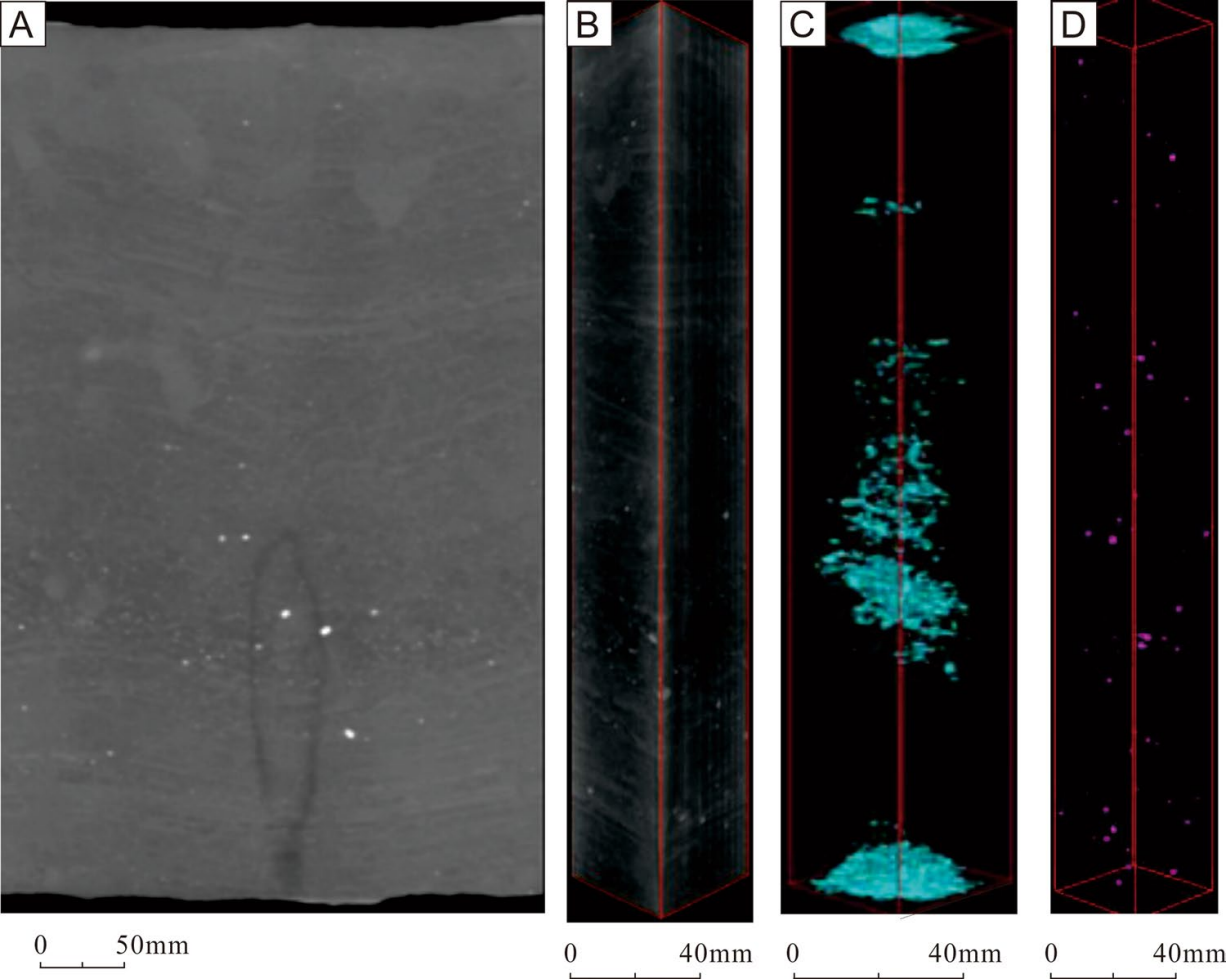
Interior structure reconstructed by ICT analysis. (A) ICT Circumferential View; (B) Images reconstructed by computed tomography; (C) 3-D Volume-rendering of open fractures (colored cyan); (D) 3-D Volume-rendering of calcite-filled fractures.
